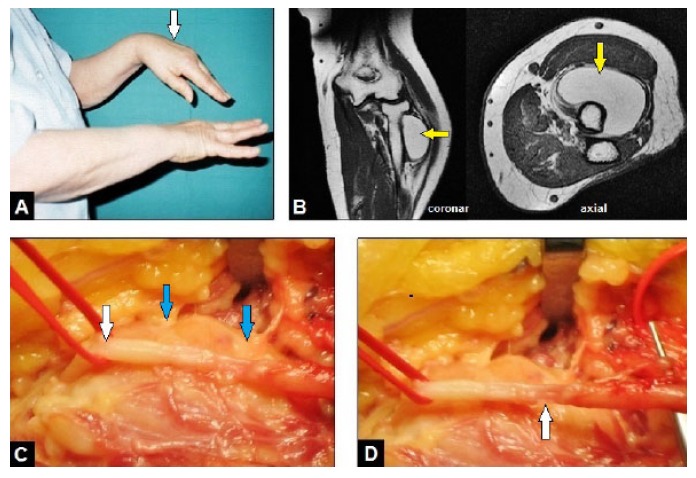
(Case presentation, initial and intraoperative findings according to the first procedure): (A) Clinical photograph of both forearms showing left (arrow) complete loss of wrist and thumb's extension and incomplete loss of extension in MCP joints II-V; (B) MRI demonstrating the monstrous GL (arrows) which surrounds the proximal radius shaft approximately a half part of its total circumference; (C) Clinical photograph showing the radial nerve (white arrow) which was fixed to the capsule of the GL (blue arrows); (D) Clinical photograph after monobloc removal of the entire GL and careful dissection of the radial nerve showing the overstretching-related partial disruption of the nerve with a gap of 1 cm involving a half part of its overall circumference (arrow).
